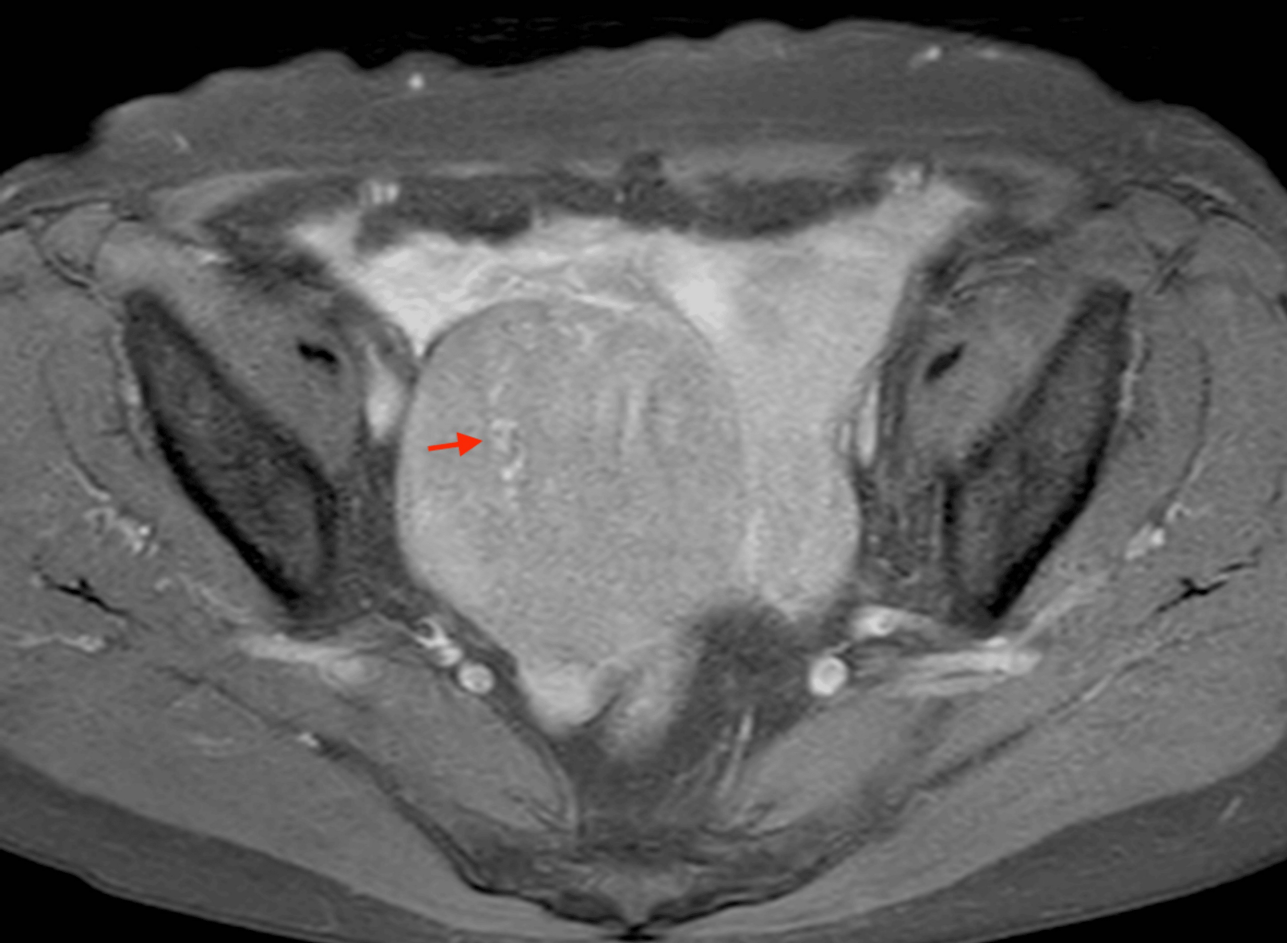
This is the same patient as shown in Figure 4. The axial fat-suppressed T1-weighted image shows high signal intensity, suggestive of hemorrhage (arrow).
